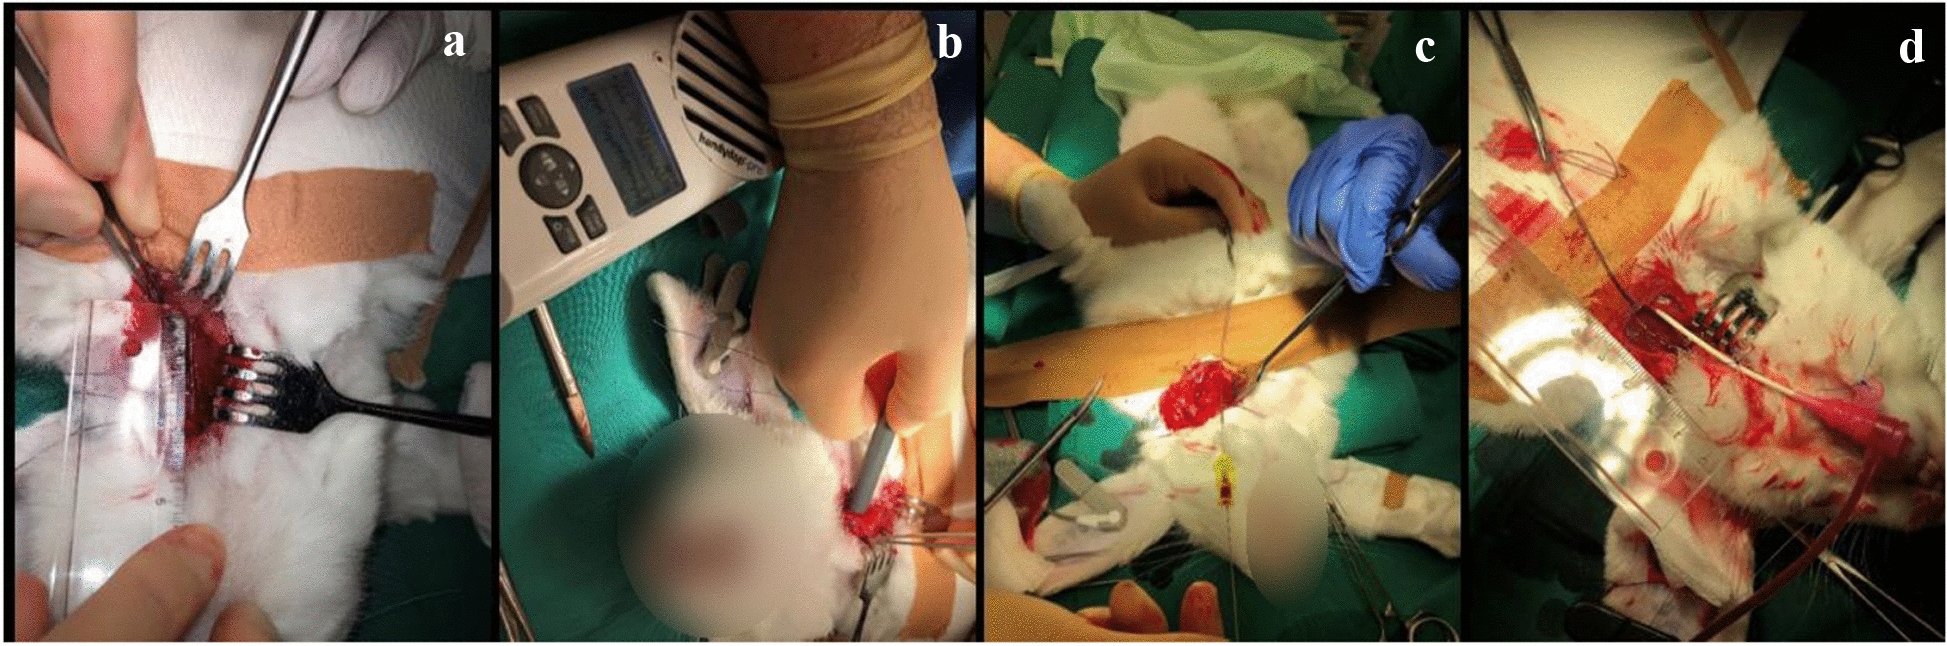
Surgical preparation of the left carotid artery of the New Zealand white rabbit for cardiac intervention. a Dissected, isolated, and fixed carotid artery. b Verification of the artery by Doppler sonography. c Puncture of the carotid artery by a Seldinger cannula and induction of the 0.014-in. coronary wire. d Fixation of the inserted 4-Fr introducer sheath
